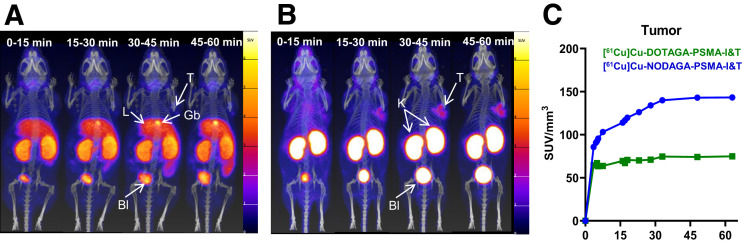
(A and B) Maximum intensity projections of dynamic PET/CT scans of LNCaP xenografts after injection of 400 pmol/7–8 MBq [^61^Cu]Cu-DOTAGA-PSMA-I&T (A) and [^61^Cu]Cu-NODAGA-PSMA-I&T (B) 0–1 h after injection, in 15-min frames. (C) Time–activity curves of tumor derived from dynamic PET/CT scans. Bl = bladder; Gb = gallbladder; K= kidneys; L = liver; T = tumor.
